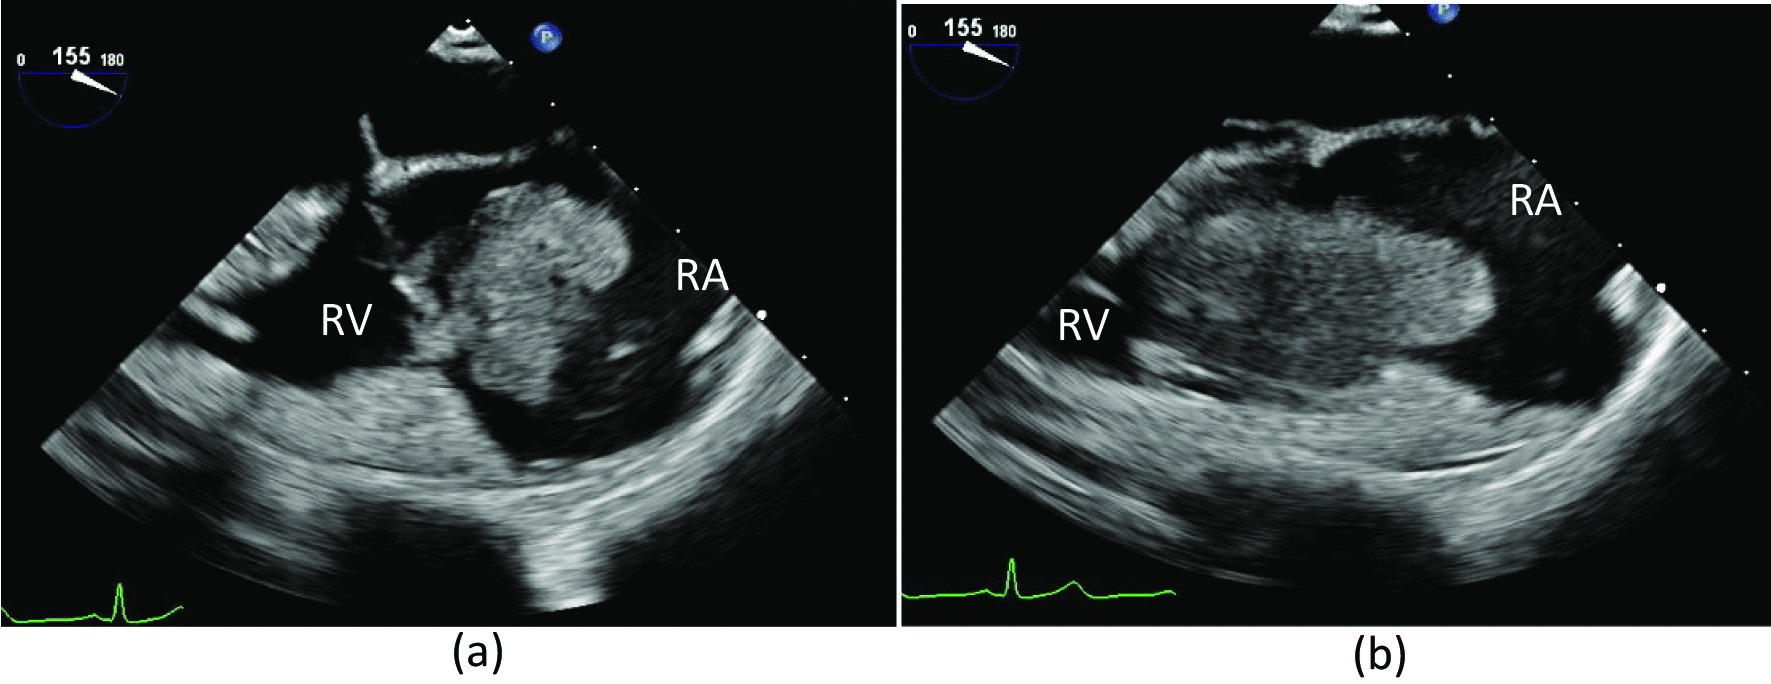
a Transesophageal echocardiography showing a large mass with heterogeneous echogenicity. b The mass passed through the tricuspid valve to the right ventricle in the diastolic phase
